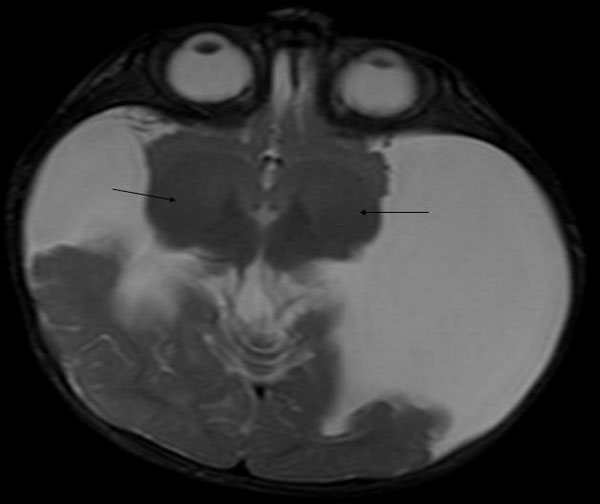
Axial T2-weighted MRI image showing bilateral inferiorly located thalami (arrows) and giant schizencephaly clefts.
